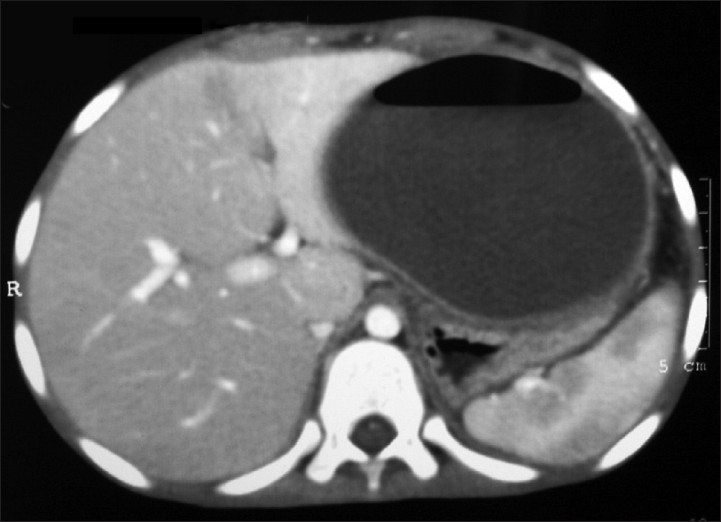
Axial view of computed tomography scan abdomen showing an air-fluid level containing cavity in between liver and stomach
